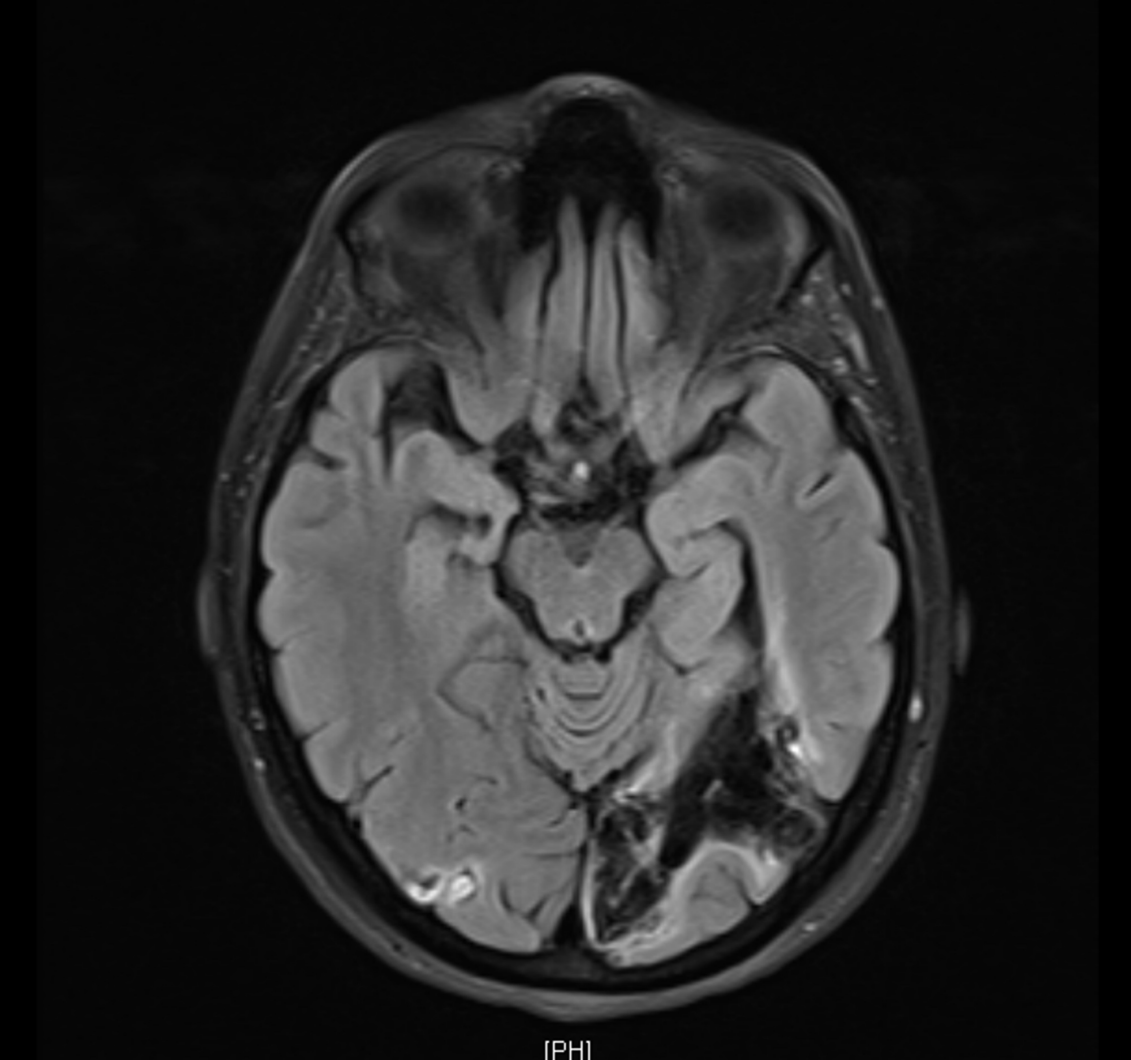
Follow-up magnetic resonance imaging (MRI) three months after discharge, showing loss of volume and encephalomalacia in the right and left occipital region representing sequelae of previous infarction
